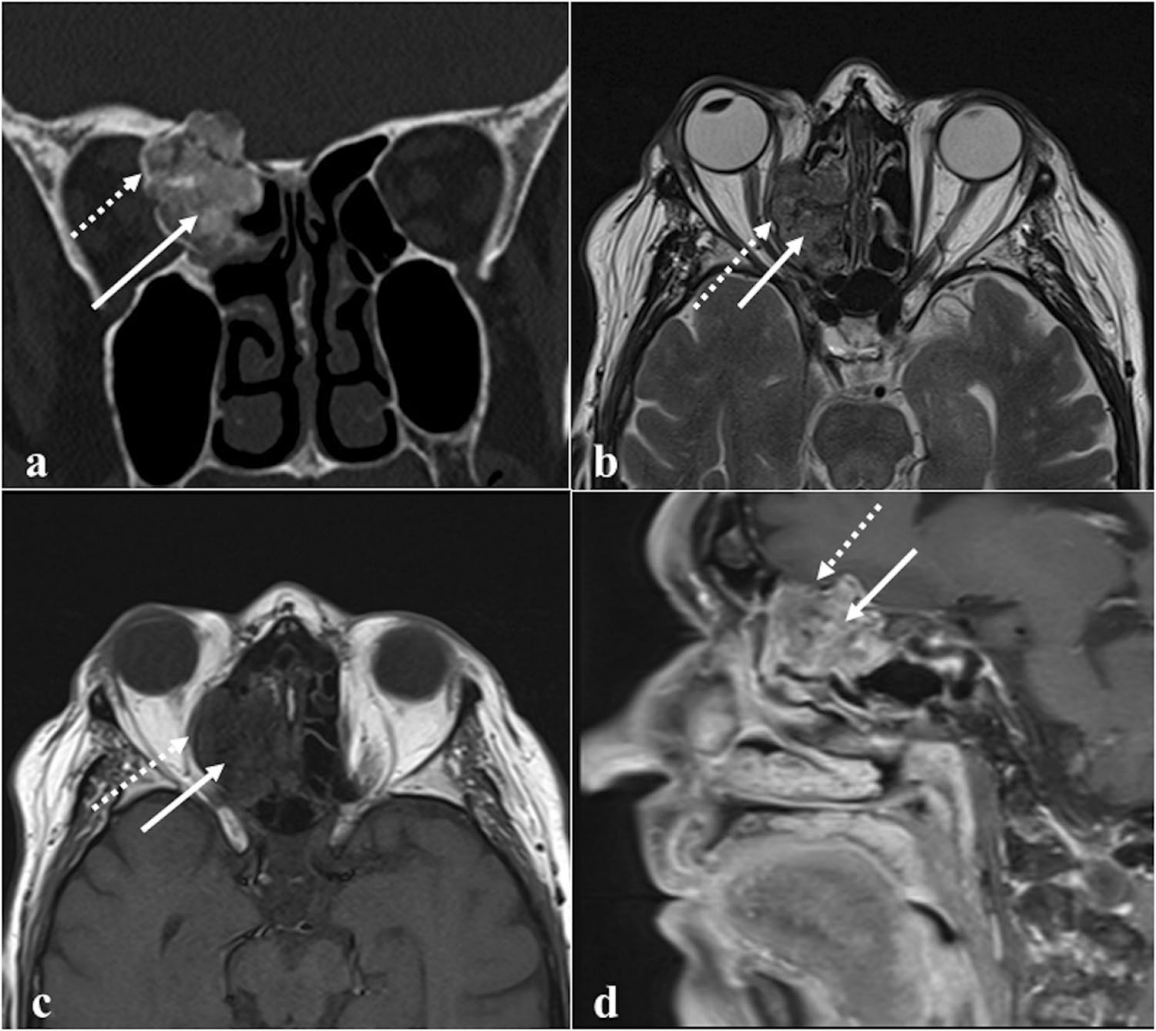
Right spheno-ethmoidal ossifying fibroma as an incidental finding in a 79-year-old female patient. Coronal CT image (a) shows a well-demarcated expansile lesion with central fibrous density areas (white arrow), surrounded by an ossified rim (white dotted arrow). MRI (b, c, and d) shows a lesion with intermediate central SI (fibrous areas, white arrows) and a peripheral rim of low SI (ossified area, white dotted arrows). The central fibrous areas have low SI on axial T1W image (b), mixed SI on axial T2W image (c), and inhomogeneous SI on sagittal T1W fat-saturated CE image (d). The peripheral ossified rim and internal septa appear hypointense in all MRI sequences
